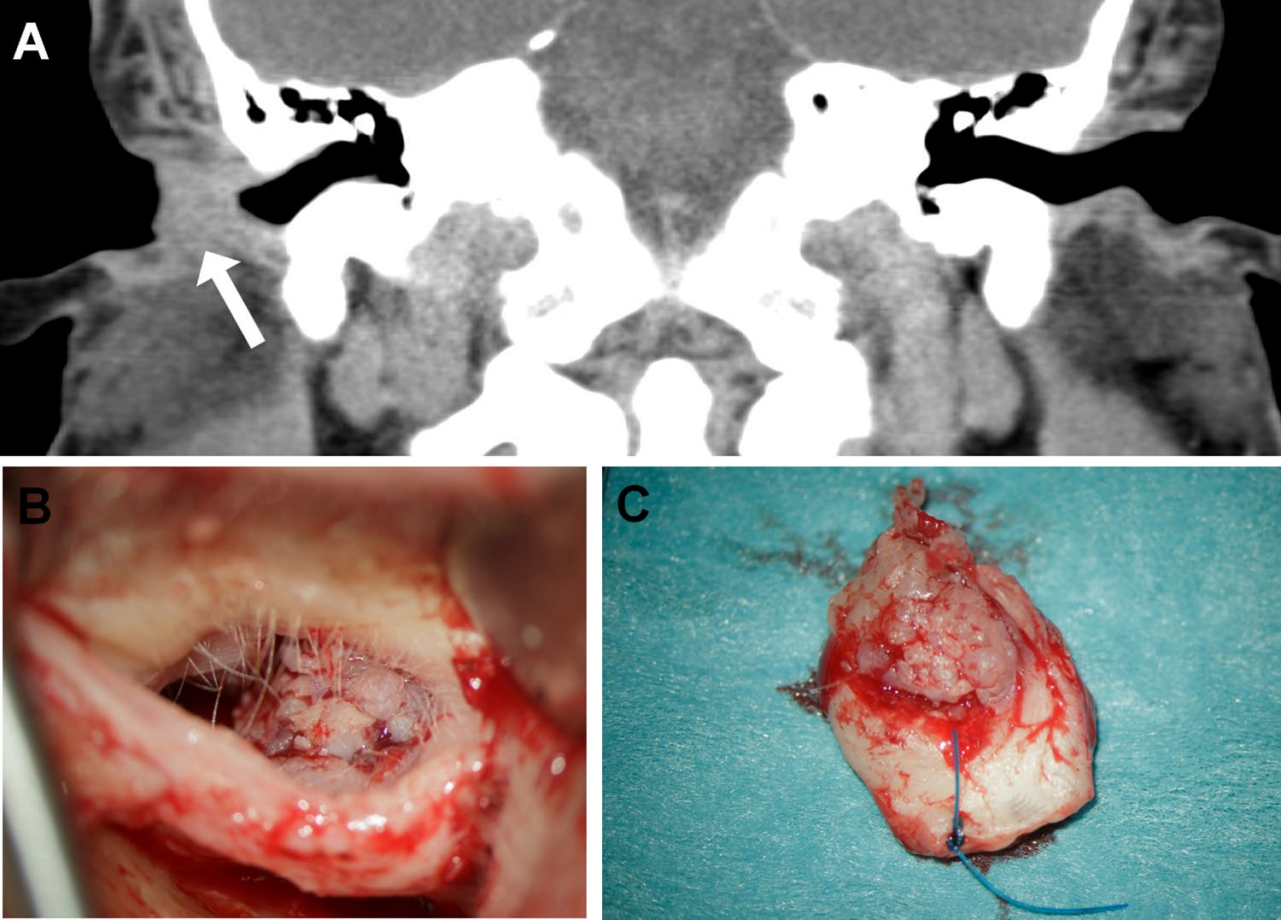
Representative examples of the appearance of the *YAP1-NUTM1*-rearranged porocarcinoma of the auditory canal on CT (a Case 1) and at surgery (b Case 2). The excised tumor in Case 2 showed a well circumscribed flat-exophytic to warty lesion (c)
